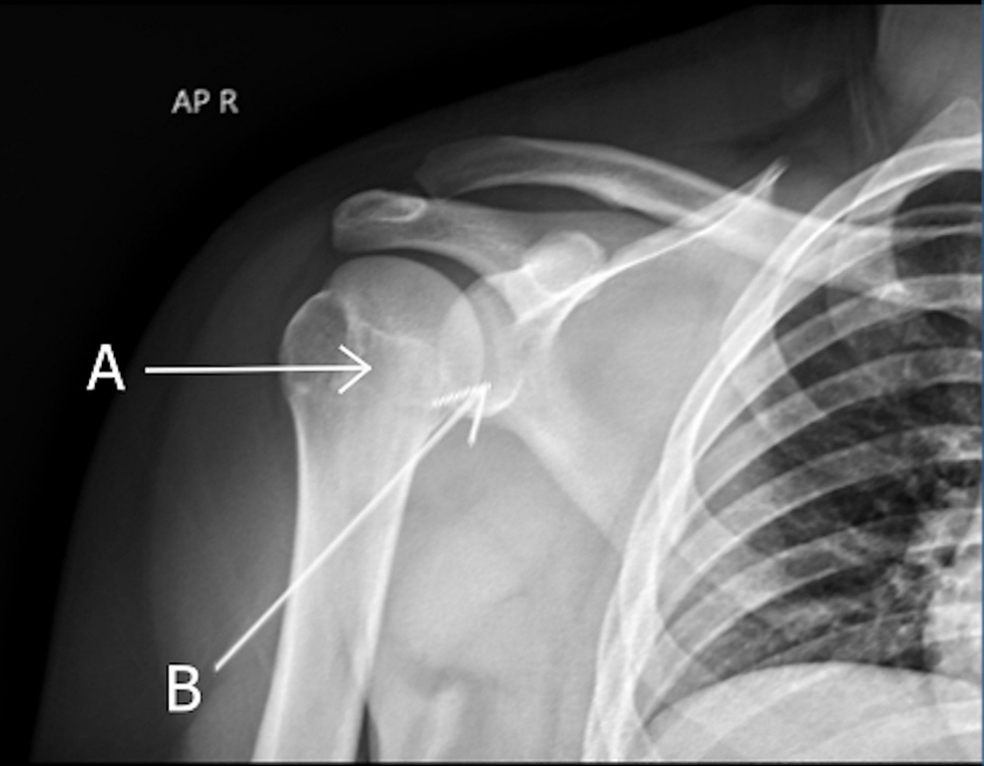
Anterior-posterior X-ray showing the right glenohumeral joint post-reduction. The humeral head (A) is back in the socket and articulating with the glenoid (B).
